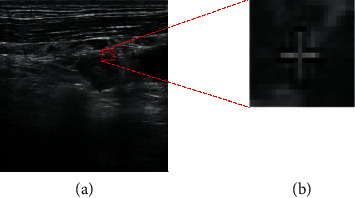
Ultrasound images of thyroid nodules with unclear artificial marks: (a) original image of having unclear artificial marks; (b) artificial marks are mixed with background pixels.
